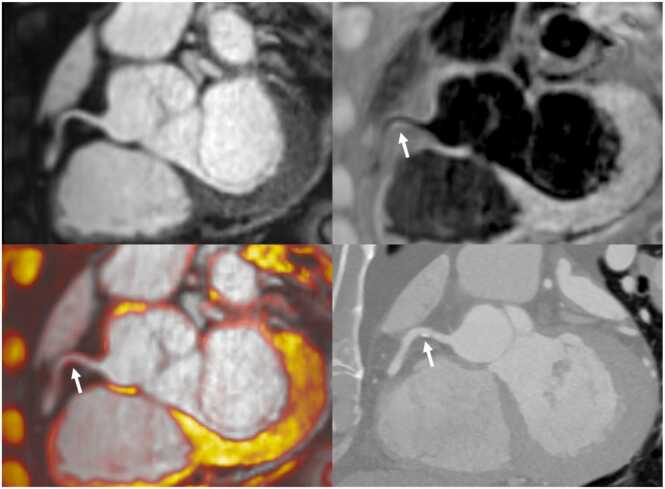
Plaque visualization in the proximal RCA using iT2prep-BOOST and CCTA. Top left: BOOST bright-blood image; top right: BOOST black-blood image; bottom left: fusion image of bright- and black-blood BOOST; bottom right: corresponding CCTA image. White arrows indicate the location of an atherosclerotic plaque in the proximal RCA, which appears hyperintense on the black-blood image and corresponds to a partially calcified lesion on CCTA. *RCA* right coronary artery, *CCTA* coronary computed tomography angiography, *BOOST* bright‐blood and black‐blOOd phase SensiTive
